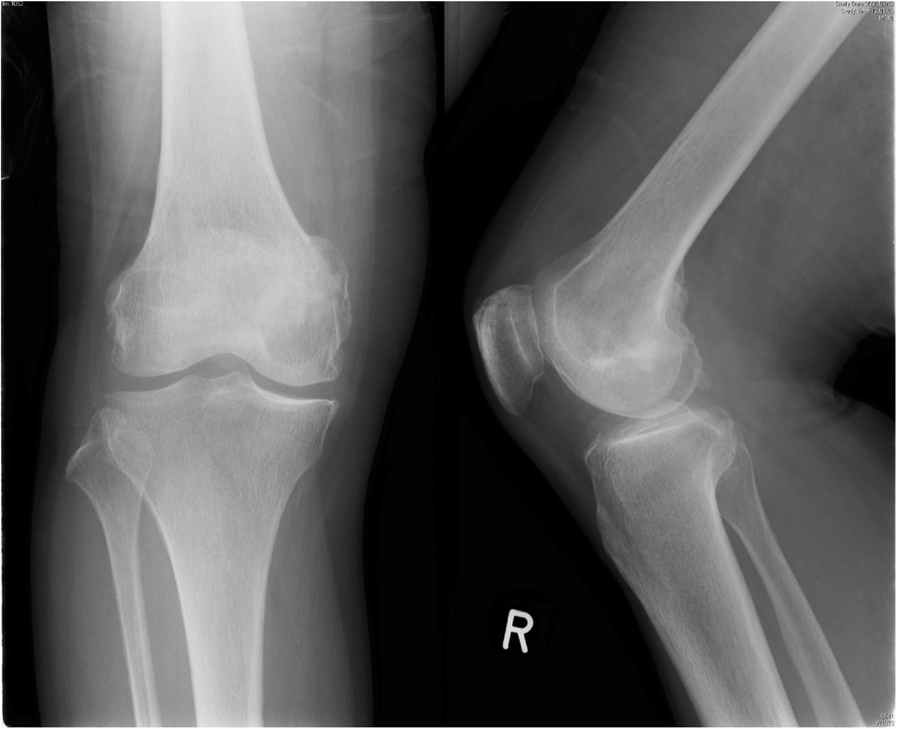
Plain radiographs of the tumor in the distal femur. Anteroposterior and lateral radiographs show a 4×4.5 cm osteolytic lesion in the right medial femoral condyle
